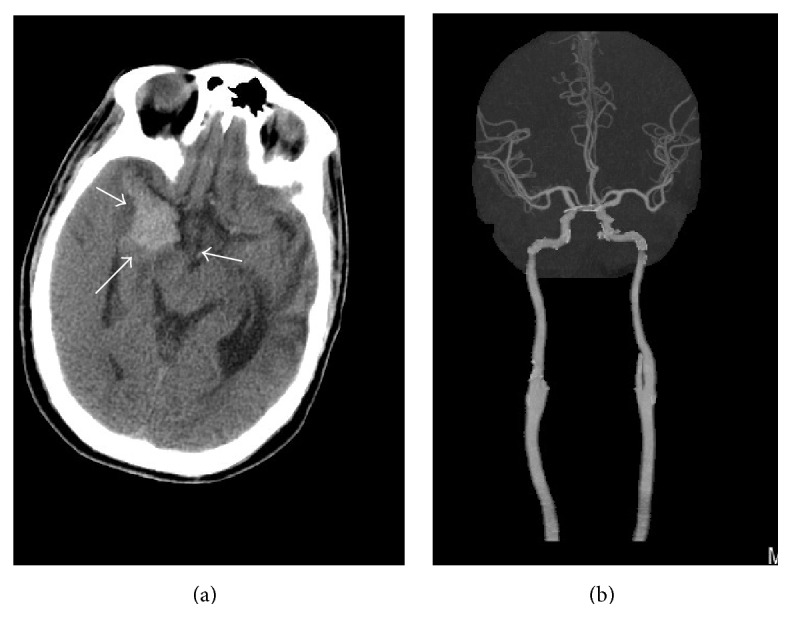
(Day 12) (a) noncontrast CT of the brain demonstrates interval development of acute hemorrhage within medial right temporal lobe, a rare complication of herpes simplex encephalitis. (b) CT angiogram with IV contrast: coronal MIP reconstruction demonstrates patency of the intracranial circulation, with widely patent intracranial arteries, including middle cerebral arteries. Arrows in (a) refer to area of acute hemorrhage within the right medial temporal lobe.
